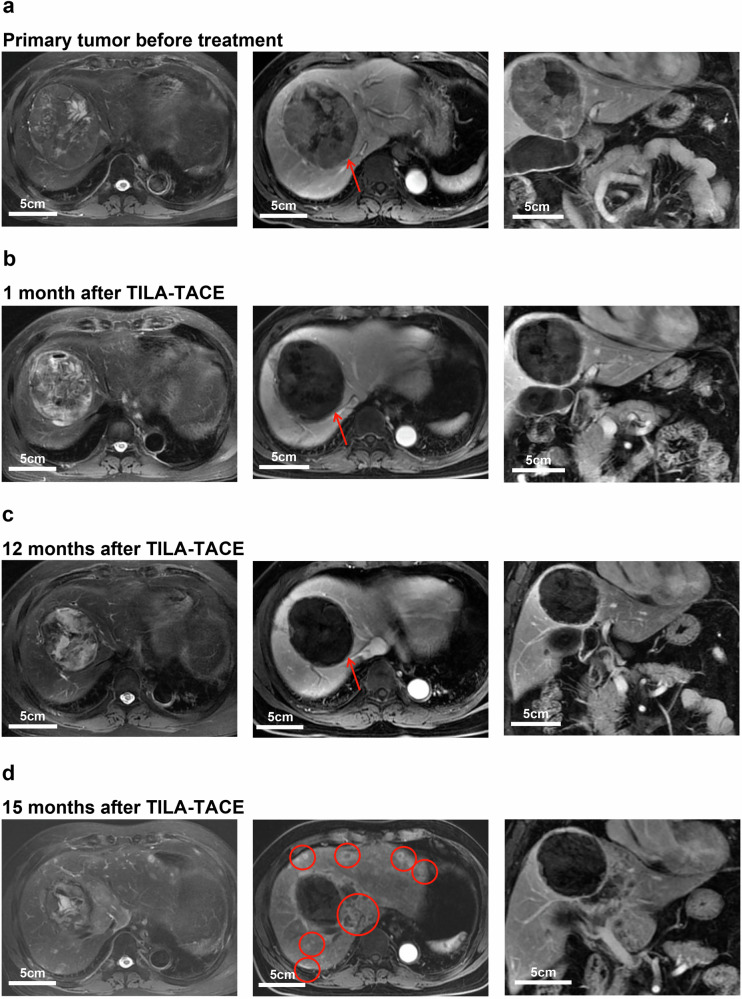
Representative MR images of subsequent intrahepatic metastasis of HCC after complete control of the targeted tumor (left: axial T2-weighted image (T2WI); middle: axial contrast-enhanced MRI; right: coronal MRI). a Scans before receiving TILA-TACE treatment, demonstrating T2 hyperintensity of the lesion (red arrow) and hyperenhancement on contrast-enhanced MR image compared with the background liver. b Scans one month after TILA-TACE treatment, demonstrating a complete necrotic lesion (red arrow). c Scans 12 months after TILA-TACE treatment, showing no new lesions or recurrences. d Scans 15 months after TILA-TACE treatment, showing that multiple intrahepatic metastases appeared (red circles)
